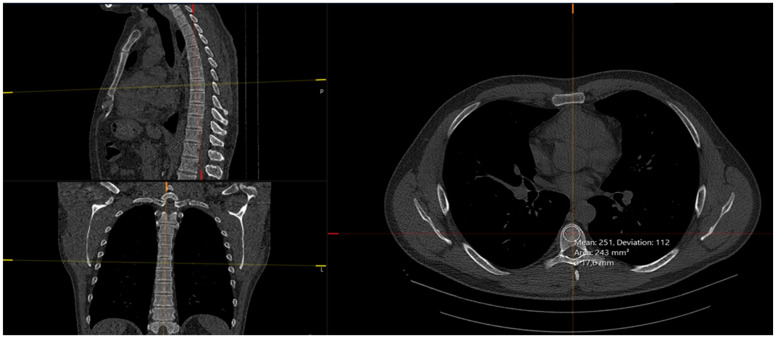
Axial, coronal, and sagittal chest CT scan in bone window representing measurement procedure of bone density with all planes placed perpendicular to measured vertebral body and ROI positioned in upper part of vertebral body.
